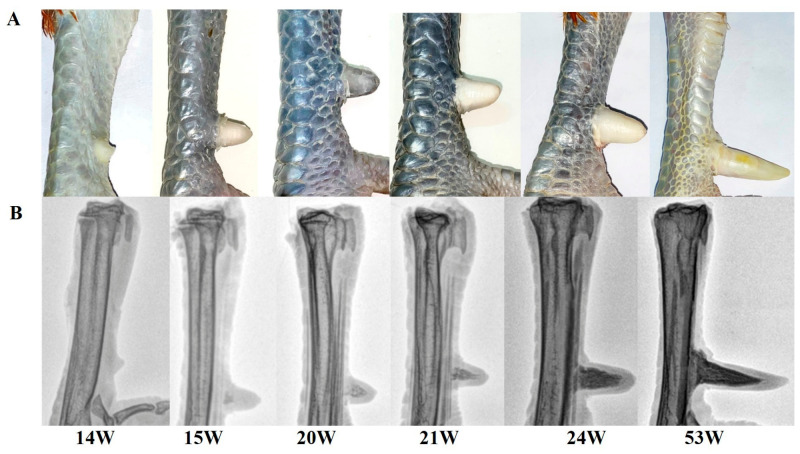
The X-ray photos of rooster spur in different times. (A) white light; (B) X-Ray.
